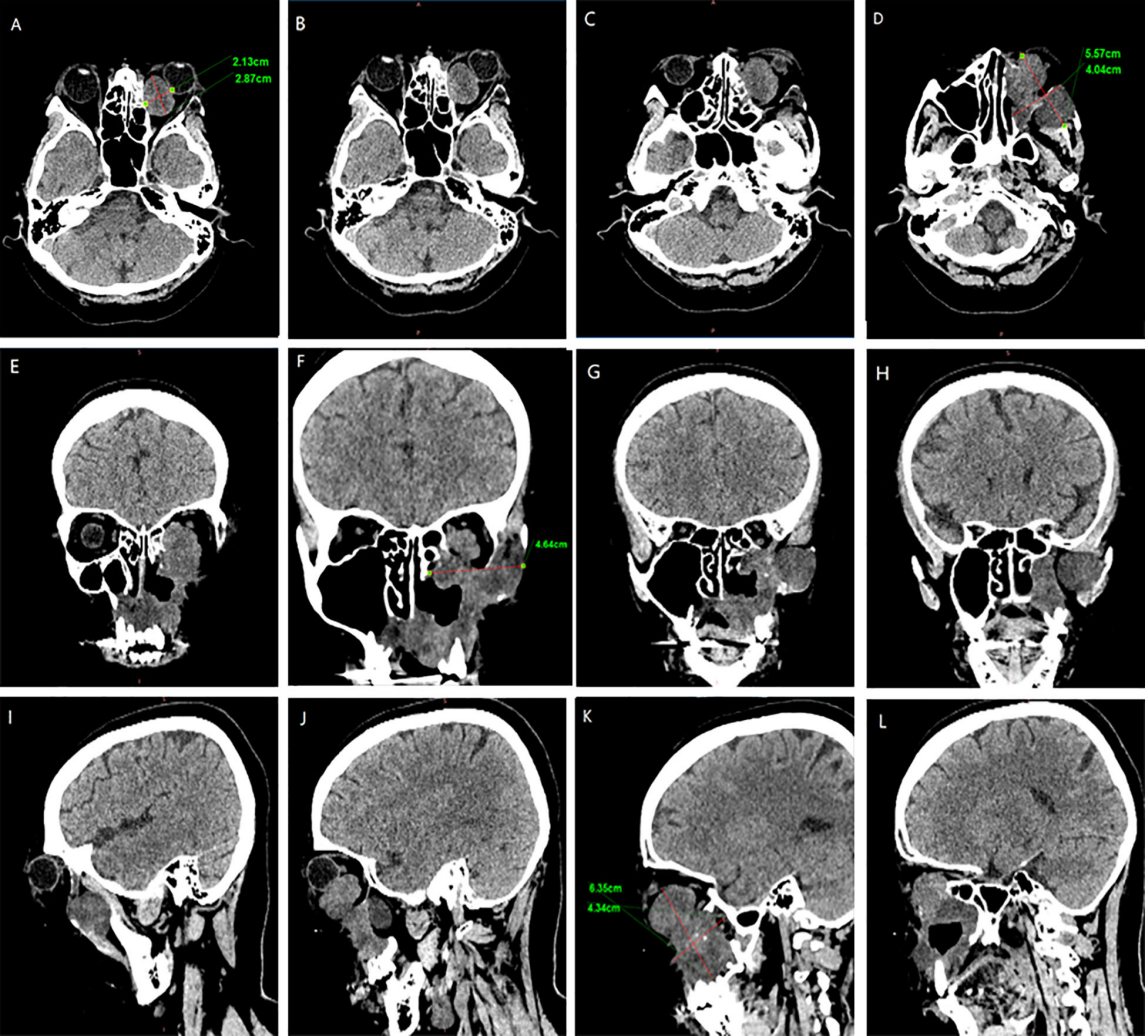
CT Anatomical imaging: Findings consistent with (LGMFS) tumor recurrence involving the orbit. (A–D) Axial CT image. (E–H) Coronal CT image. (I–L) Sagittal CT image.
